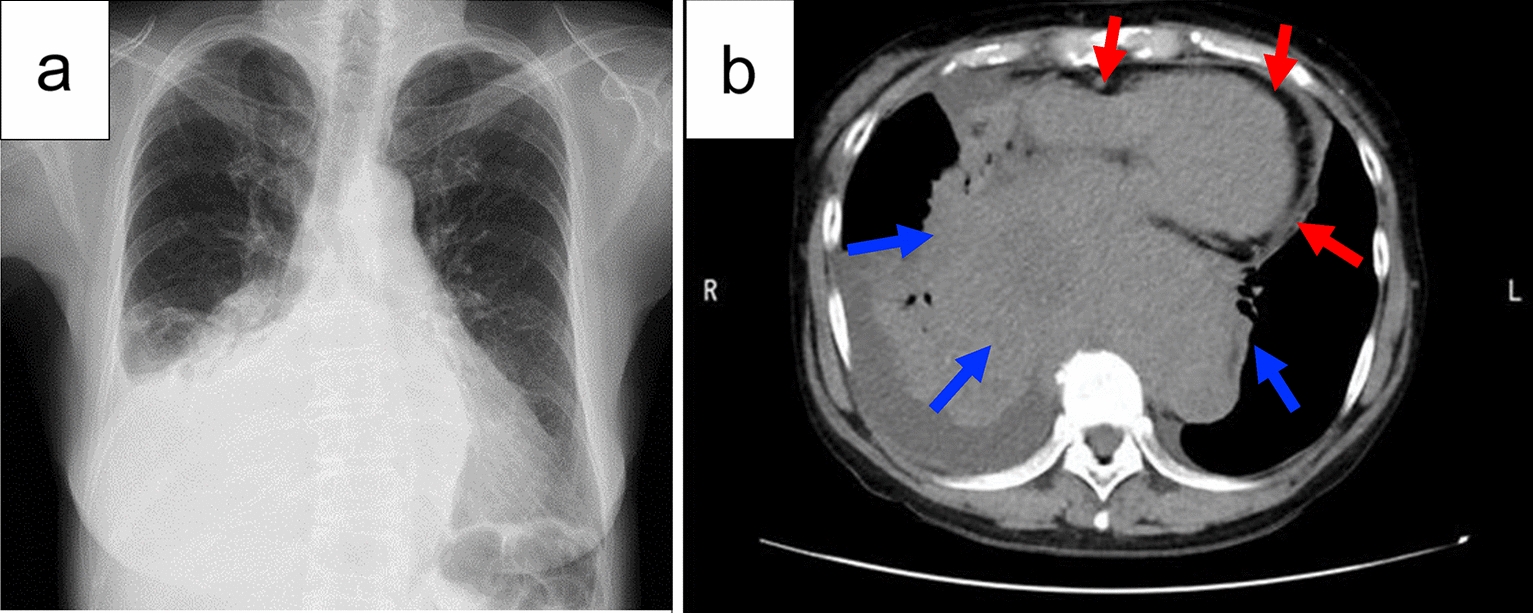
Chest X-ray and CT scan at diagnosis. a Chest X-ray showing atelectasis of the middle and lower lobes of the right lung. b Axial computed tomography showing a posterior mediastinal mass with a diameter of 12 cm (blue arrows) compressing the heart (red arrows). *CT* computed tomography
